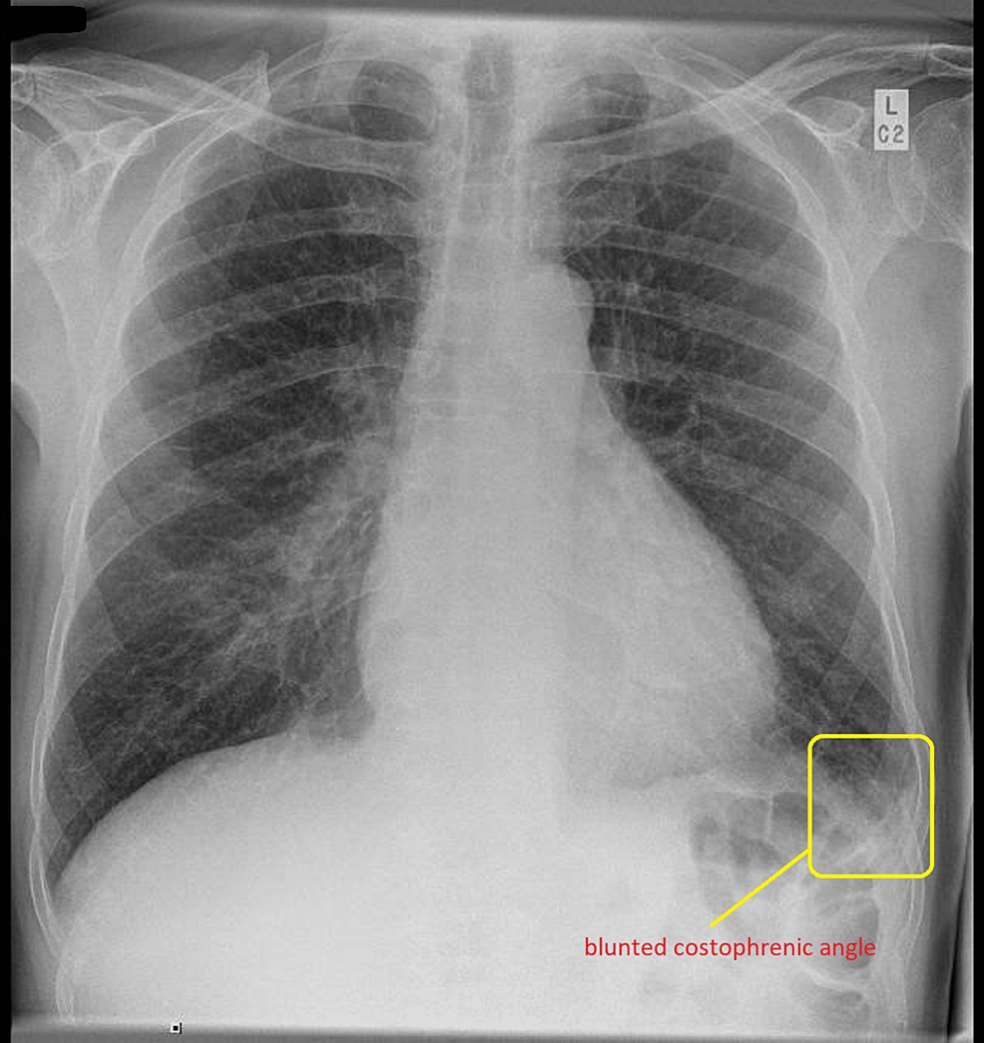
Chest X-ray Chest X-ray showing mildly blunted left costophrenic angle suggestive of small pleural effusion
